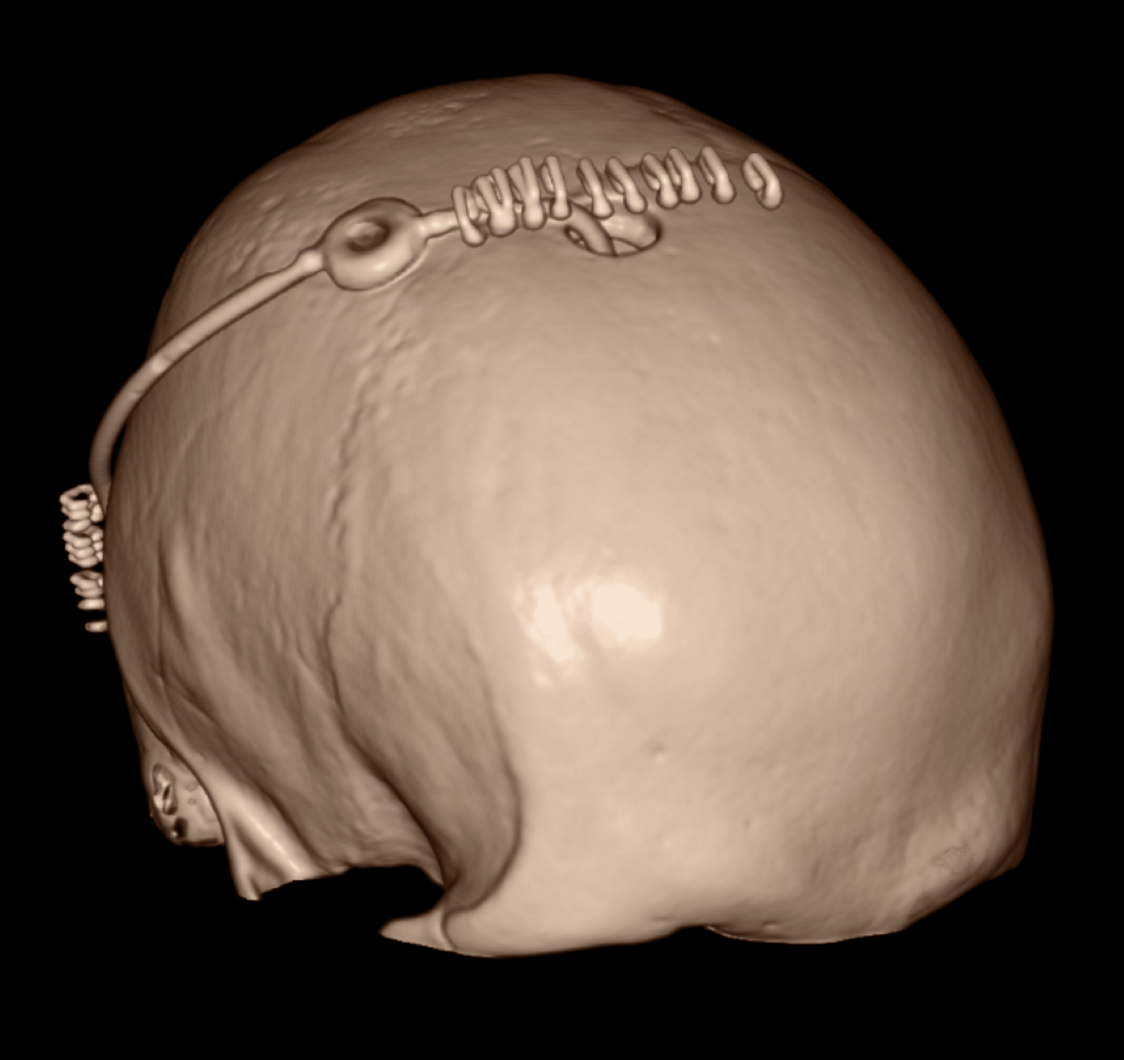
CT of the head following the insertion of the ventriculoperitoneal shunt.
